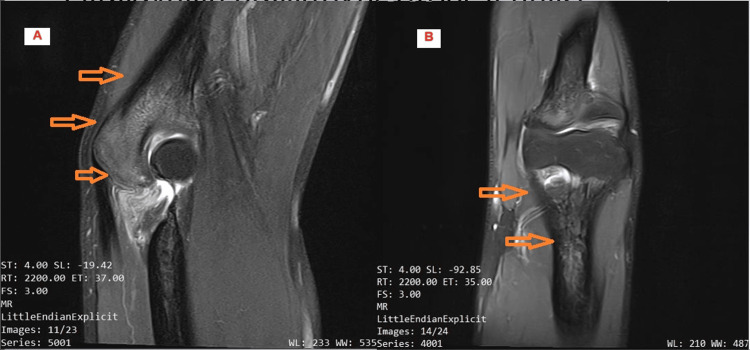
Second MRI. Sagittal (A) and coronal (B) views on persistent bone marrow oedema (arrows) three months after the first MRI and after conservative treatment.
